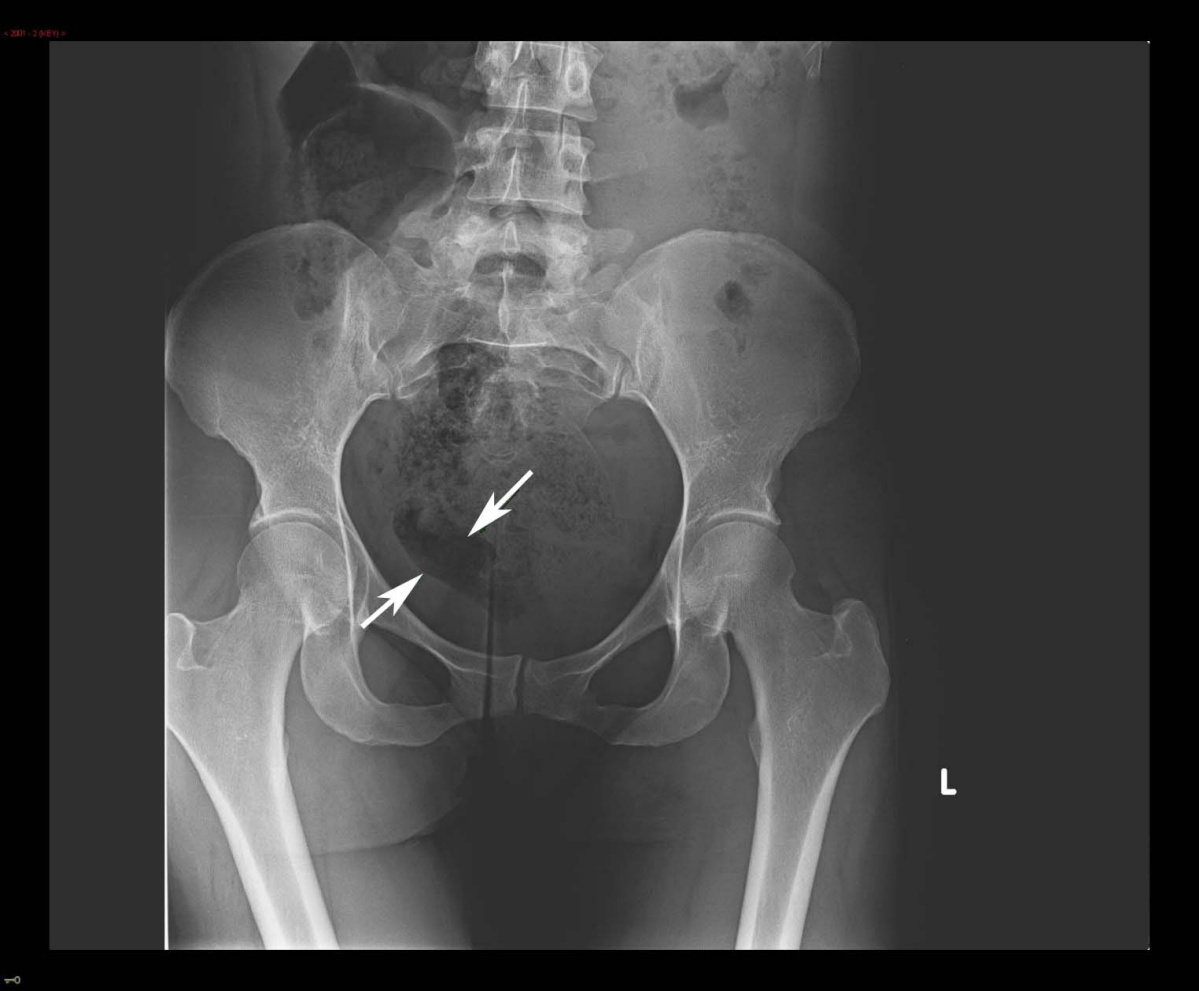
Kidney, ureter, bladder radiograph with arrows showing an area concerning for a possible foreign body in the right hemipelvis
